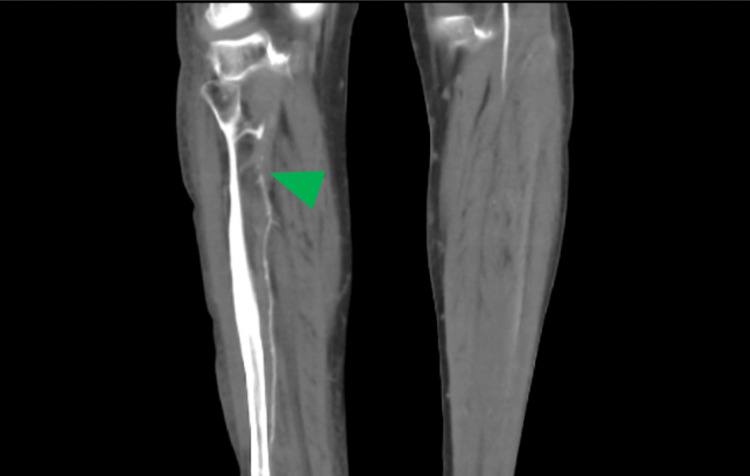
CT angiogram showing chronic long-segment stenosis of the anterior and posterior tibial arteries (green arrow), with acute embolic occlusion of the mid-to-distal right peroneal artery.
